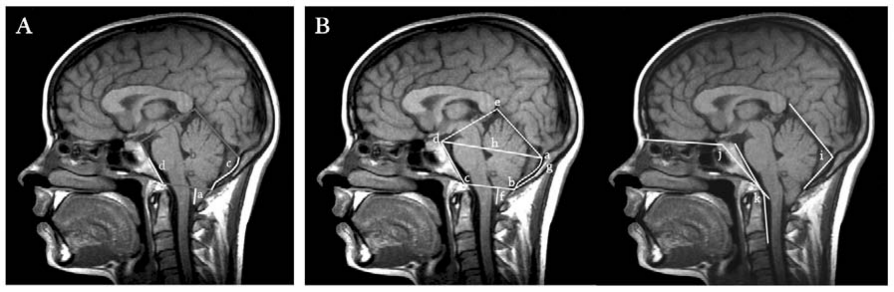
Typical neuroradiological findings in CMI. A) T1-weighted mid-sagittal MR image showing downward herniation of the cerebellar tonsils (a) and hypoplasia of the occipital components of the posterior cranial fossa (b): the supraocciput (c) and the basiocciput (d). B) Morphological measurements performed: length of tonsillar descent (f), supraocciput (g-b) and clivus (c–d) and foramen magnum diameter (b–c). The antero-posterior diameter of PCF (h) was inferred from a line running from the internal occipital protuberance (a) to top of the dorsum sellae (d). The PCF area was estimated as the polygon delimited by (a) (b) (c) (d) and (e) and the osseus PCF area as the one delimited by (a) (b) (c) and (d). Angular measurements: tentorium angle (i), basal angulation (j) and Wackenheim angle (k).
